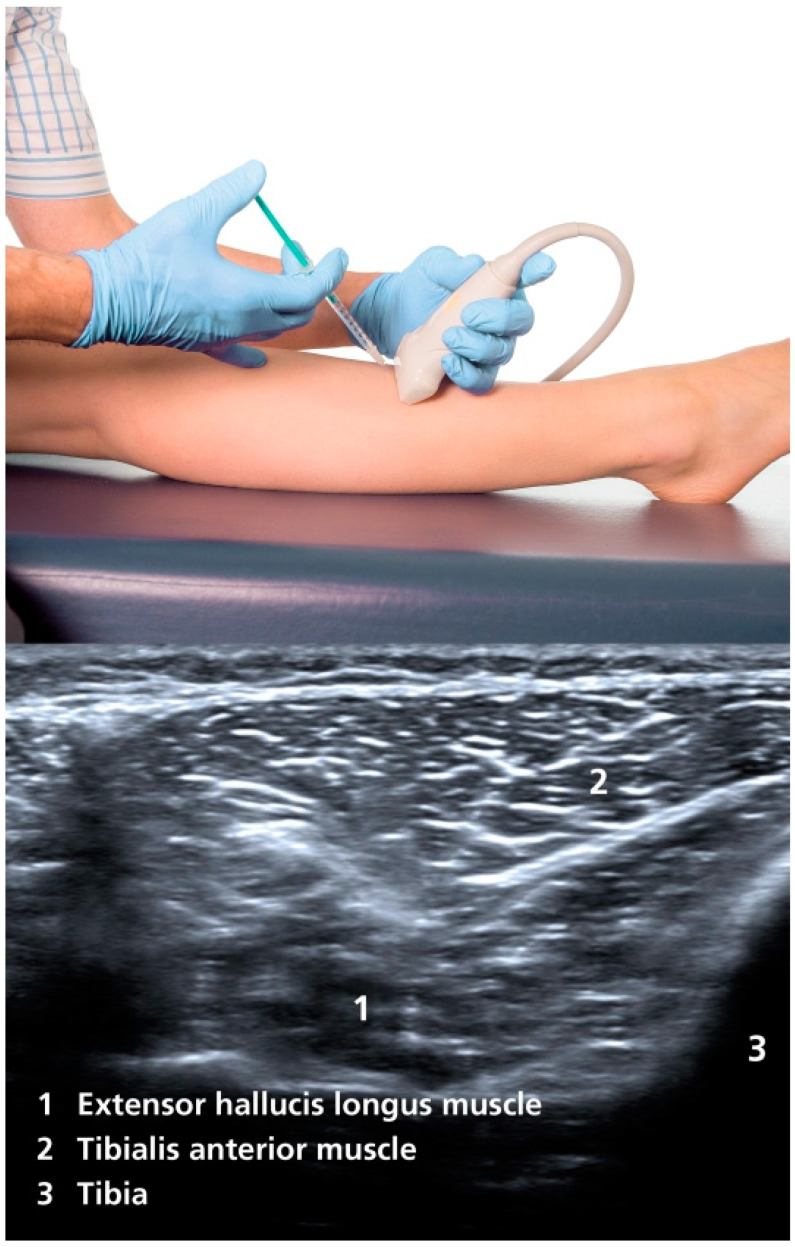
Injection site and ultrasound imaging of the extensor hallucis longus muscle [21].
